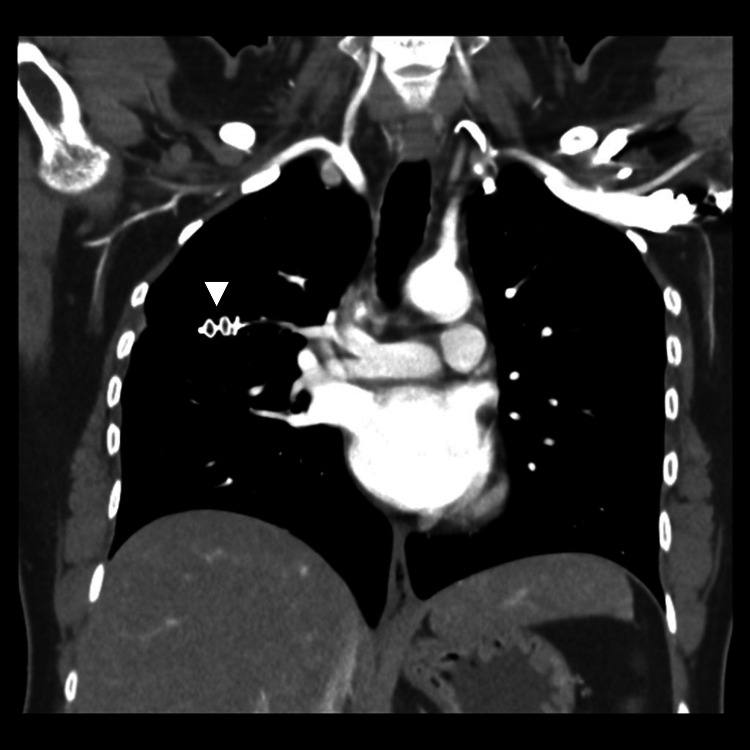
Coronal CT angiogram images done on two-month follow-up showing a vascular Amplatzer plug in place (white arrowhead) without visualization of any residual filling of arteriovenous malformation.
